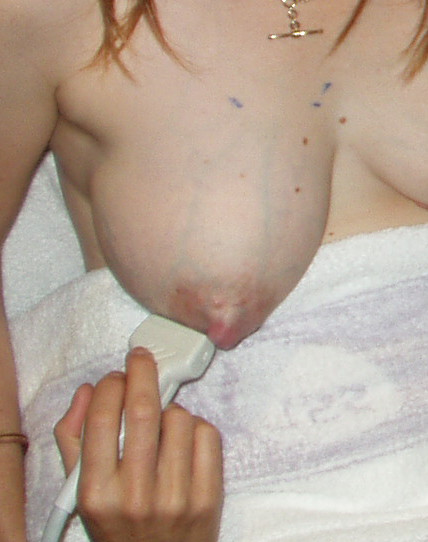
Ultrasound scanning position for detection of milk ejection in the lactating breast. The breast that is not suckled/expressed is monitored using a high frequency linear array ultrasound transducer. The milk duct monitored is in the lateral portion of the breast near the base of the nipple. Minimal pressure must be used to avoid compression of the duct.
